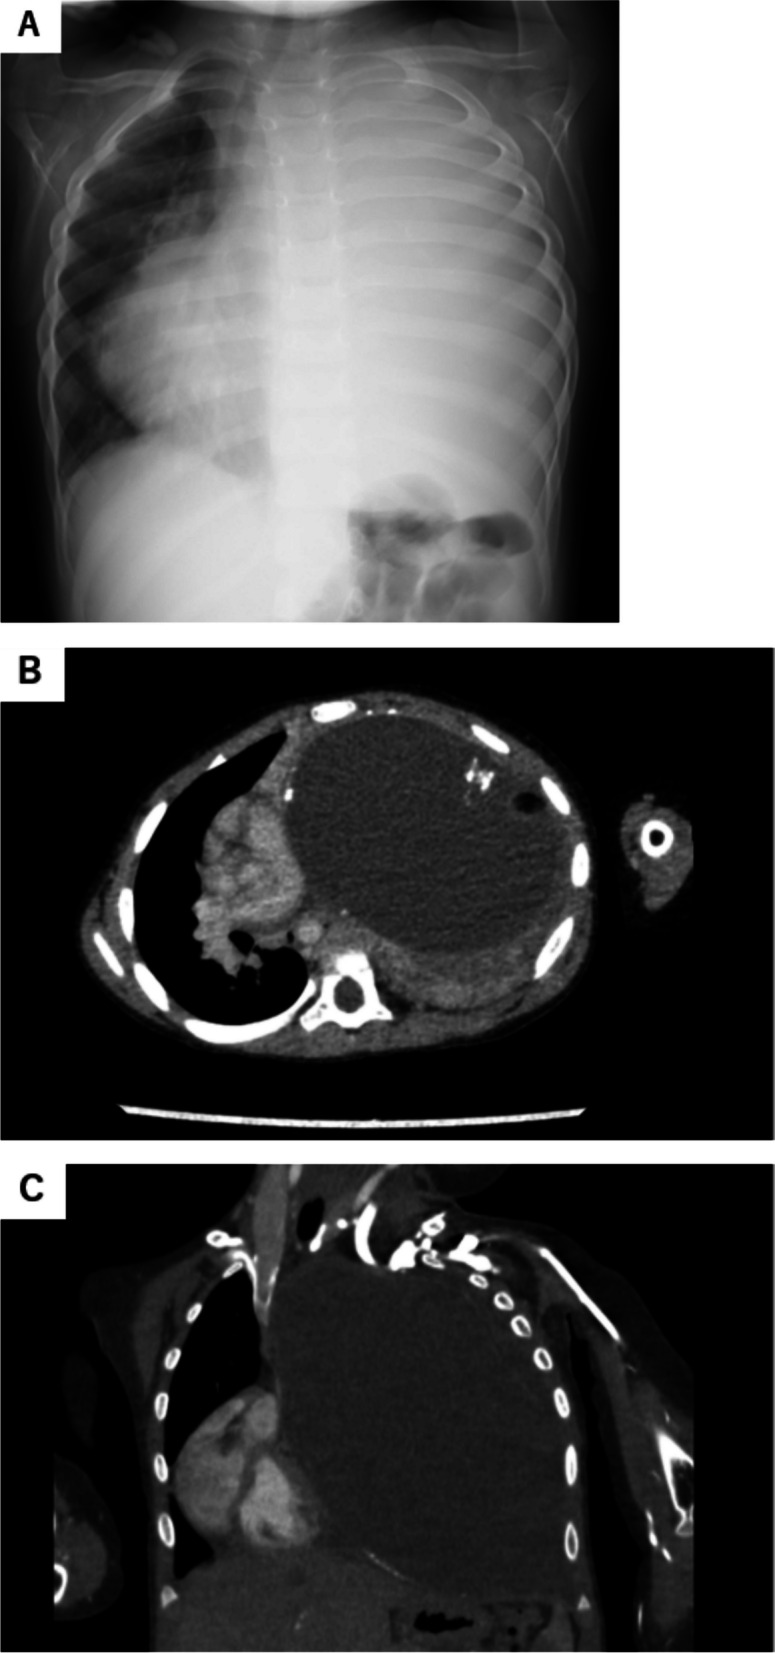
Chest radiograph (A) showing a huge tumor occupying the left thoracic cavity, and the heart shadow deviated to the right side. Chest CT showing a huge tumor, 10.5 cm in size. The tumor had calcified and adipose tissue components and completely occupied the left cavity. Images are shown from B axial and C coronal views
